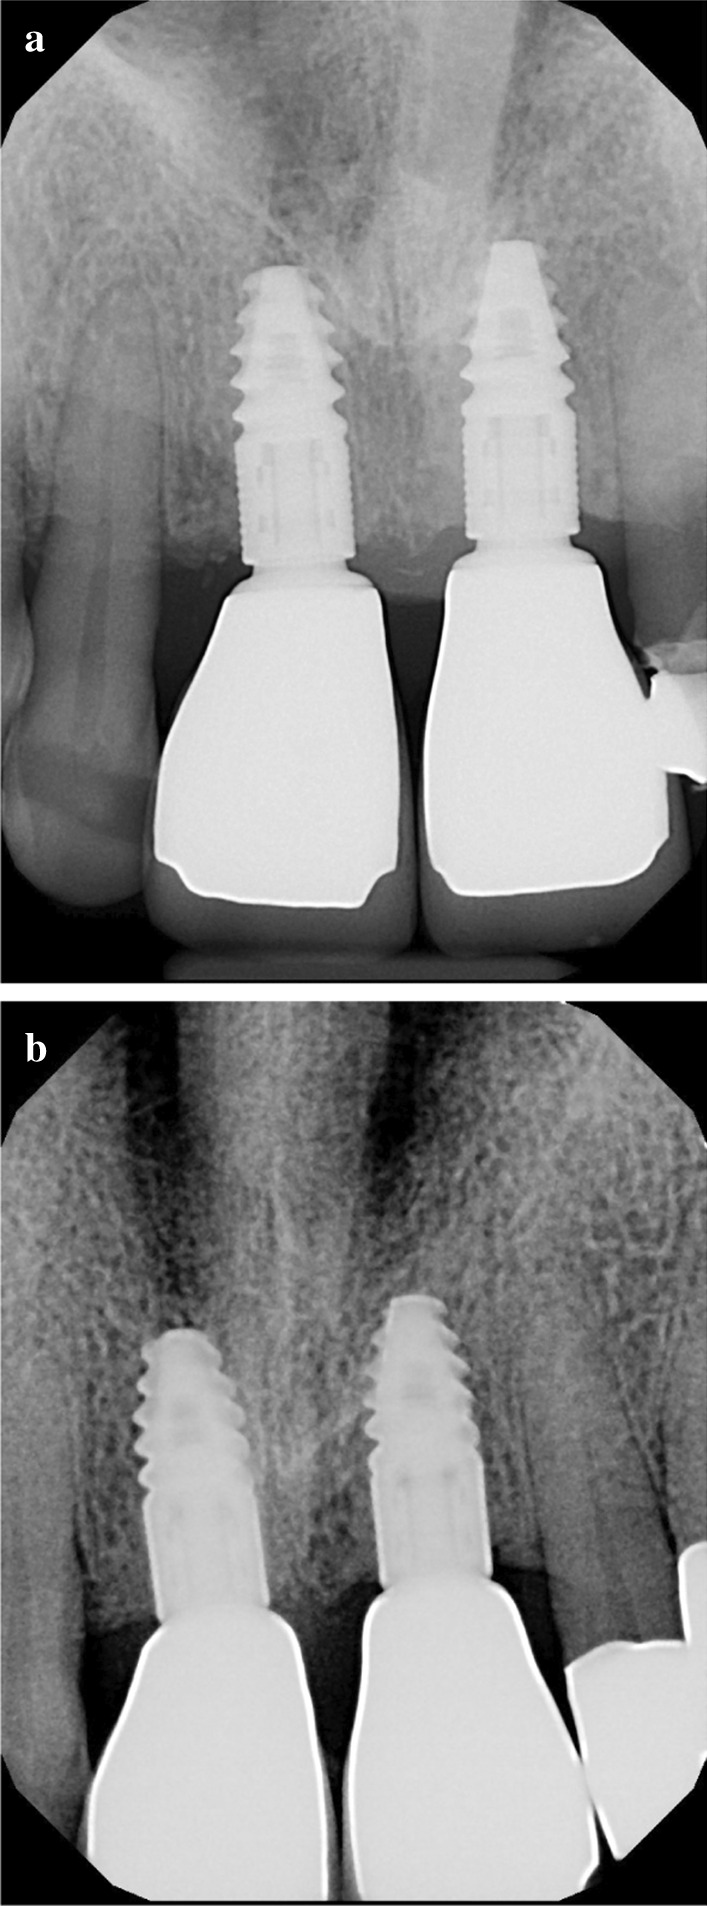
(a) Intraoral X-ray at delivery of final restorations, (b) 12 months after delivery
